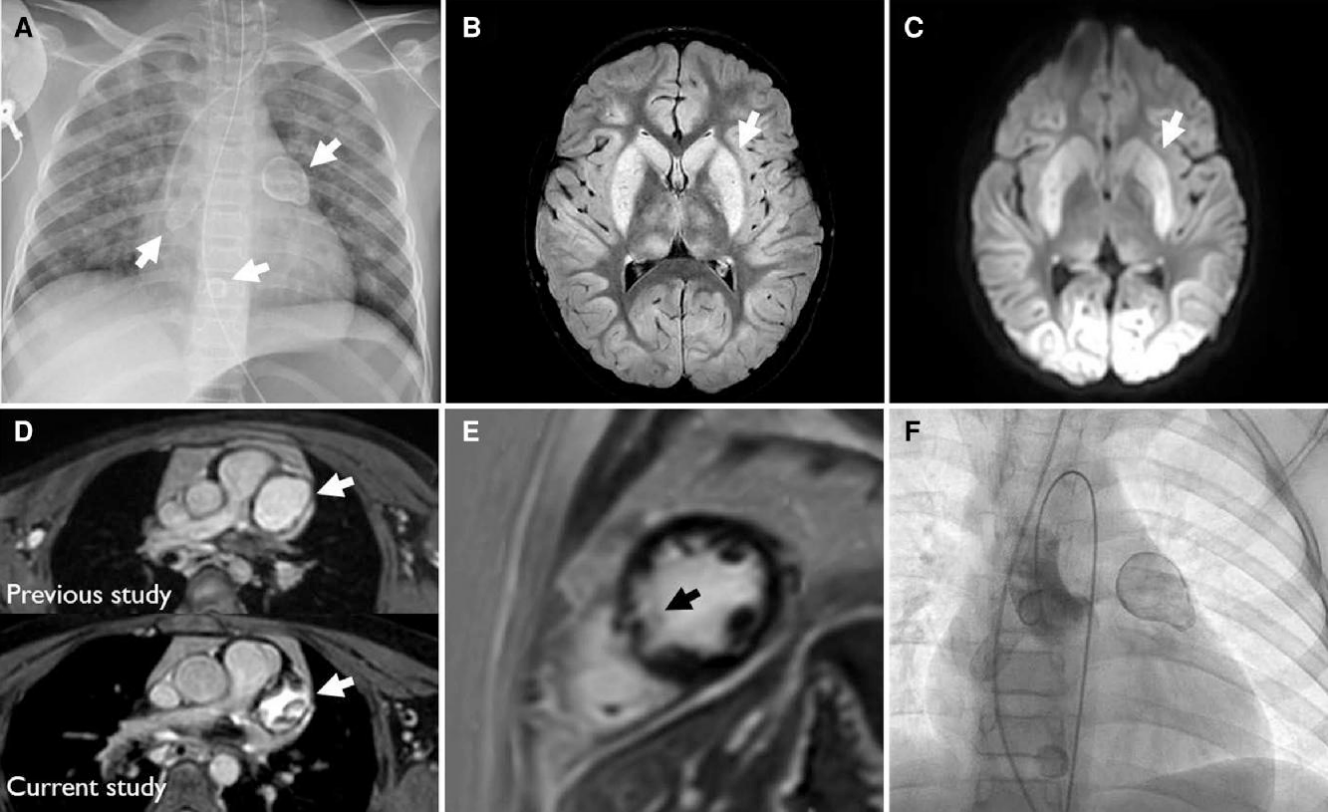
An 8-year-old female patient with Kawasaki disease presented to the emergency department with return of spontaneous circulation after 40 min of resuscitation due to ventricular fibrillation. Chest X-ray showed three giant coronary aneurysms with calcified margins in the proximal left anterior descending (LAD) and proximal and distal right coronary artery (RCA) (*Panel A*, arrows). The coronary aneurysms were already known before and were so far treated with immunoglobulin, aspirin, cortisone, and infliximab.
